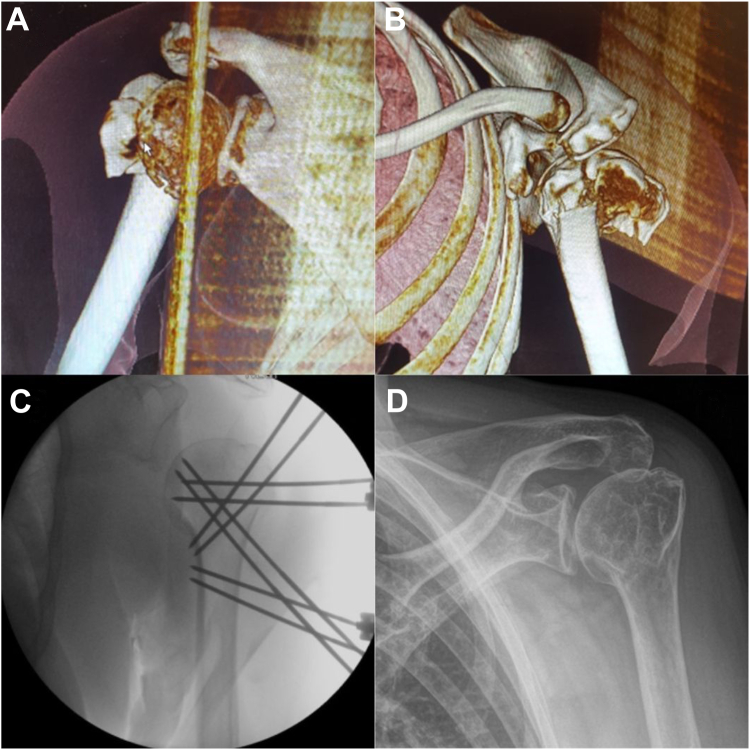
3D CT scan showing a left-sided complex Hertel type 7 proximal humerus fracture (A and B); intraoperative reduction and definitive fixation with 4 pairs of blocked threaded wires (C); final follow-up X-ray 9 years postoperatively (D). *3D*, three-dimensional; *CT*, computed tomography.
